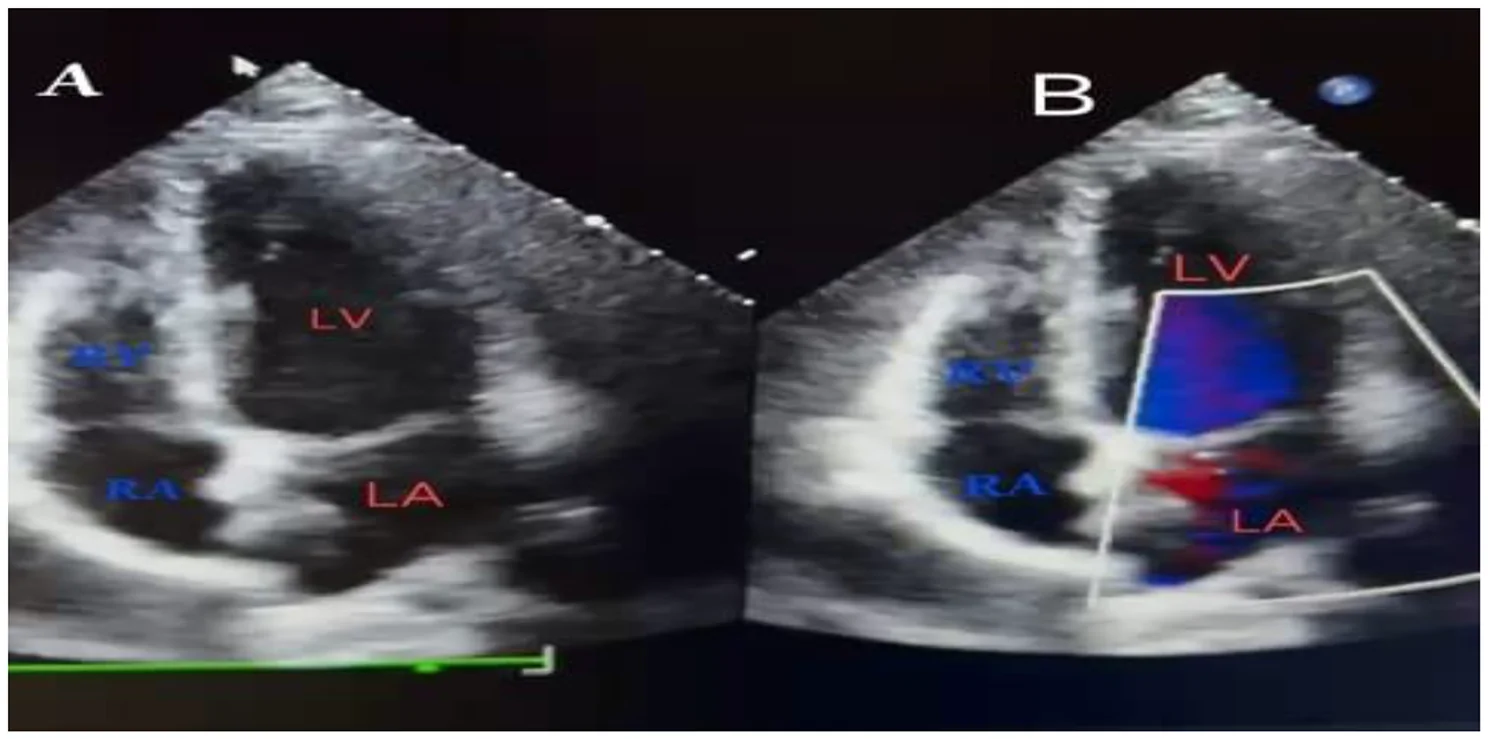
Transthoracic echocardiographic assessment following postoperative ST-segment elevation. (A) Apical four-chamber view demonstrating preserved biventricular systolic function without regional wall motion abnormalities. (B) Color Doppler imaging demonstrating no significant mitral or tricuspid valvular regurgitation and no evidence of pericardial effusion
